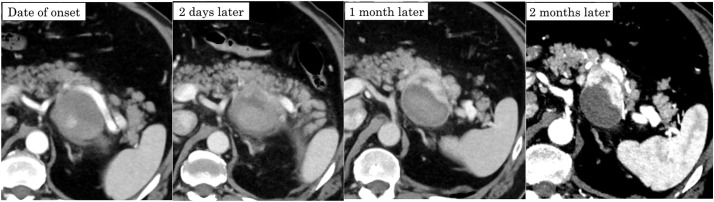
Changes in CT scan findings of the lesion over time. All images were from the arterial phase. Compared with later CT scans, the scans on the day of onset and 2 days later showed weaker contrast enhancement in the ventral area of the tumor, which was presumed to be due to vascular spasm.
